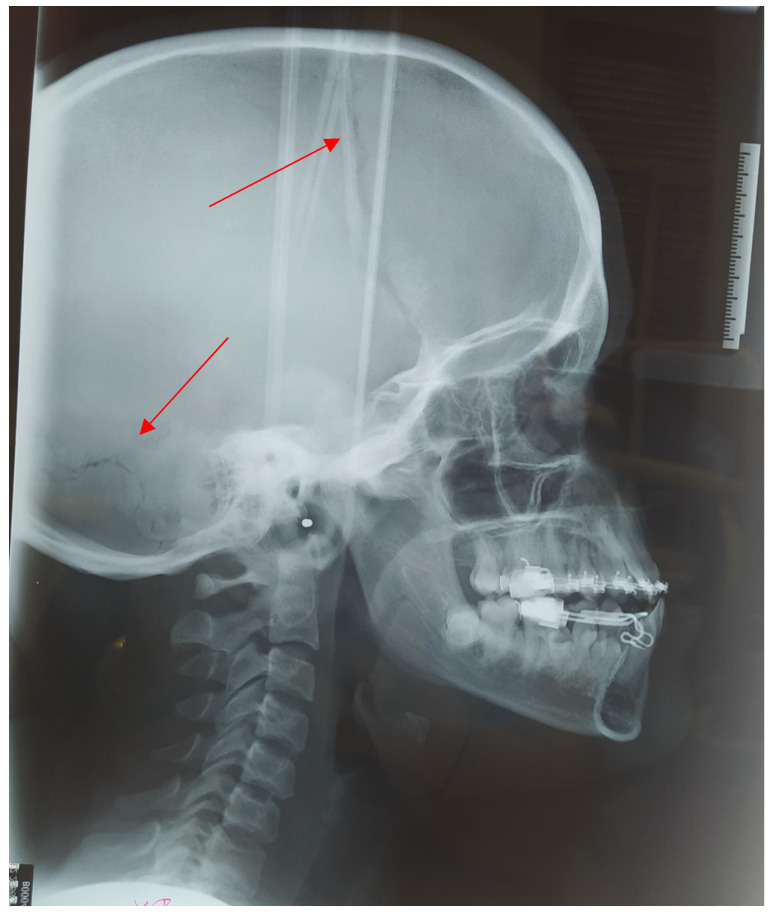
Teleradiography X-ray of sagittal plane in 2021, presence of open cranial sutures (red arrows).
